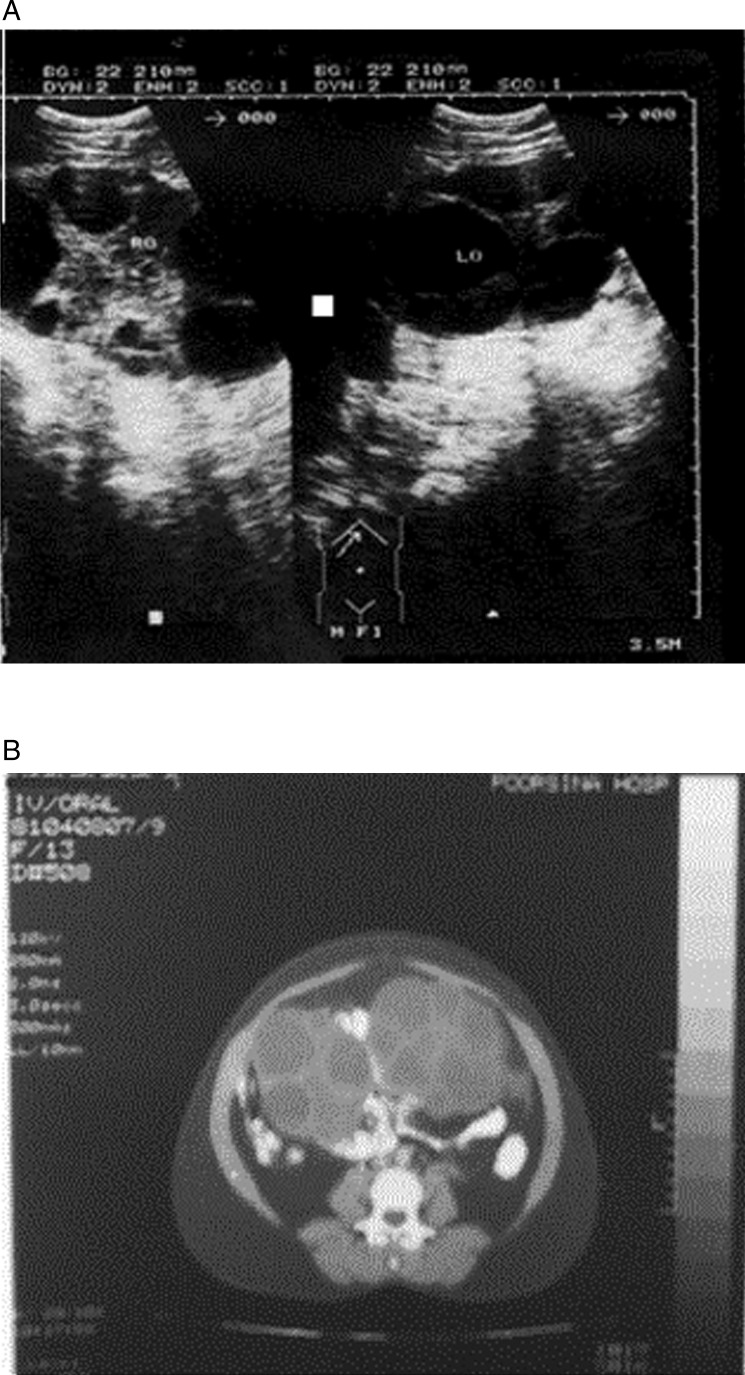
Imaging findings show bilateral multilobulated ovarian cysts. (A) Abdominal ultrasound and (B) abdominal CT scan (3).
